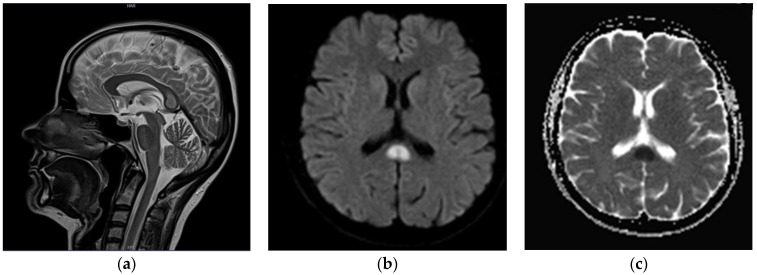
(a) T2-weighted (blade) images in sagittal plane; (b) DWI b = 1000 axial images; (c) corresponding Apparent Diffusion Coefficient (ADC) map. Well-demarcated, T2 hyperintense lesion (15 × 10 × 15 mm) within splenium of the corpus callosum (a,b) with apparent diffusion restriction on DWI/ADC images (c).
